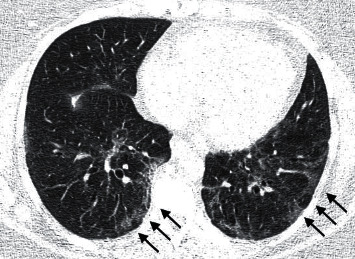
Chest CT in a lung window. An axial section at the level of the lower lobes. Subpleural ground-glass opacities (arrows).
